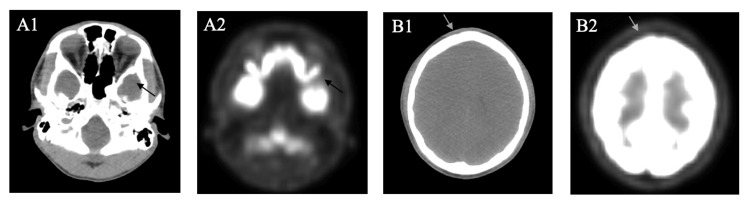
PET/CT findings after four cycles of cladribine. (A1) CT, (A2) PET: Stable size of the left sphenoid lesion (arrow) with near-resolution of abnormal FDG activity; (B1) CT, (B2) PET: Stable size of the right frontal bone lesion (arrow) with decrease in FDG activity. PET/CT, positron emission tomography/computed tomography; FDG, fluorodeoxyglucose
